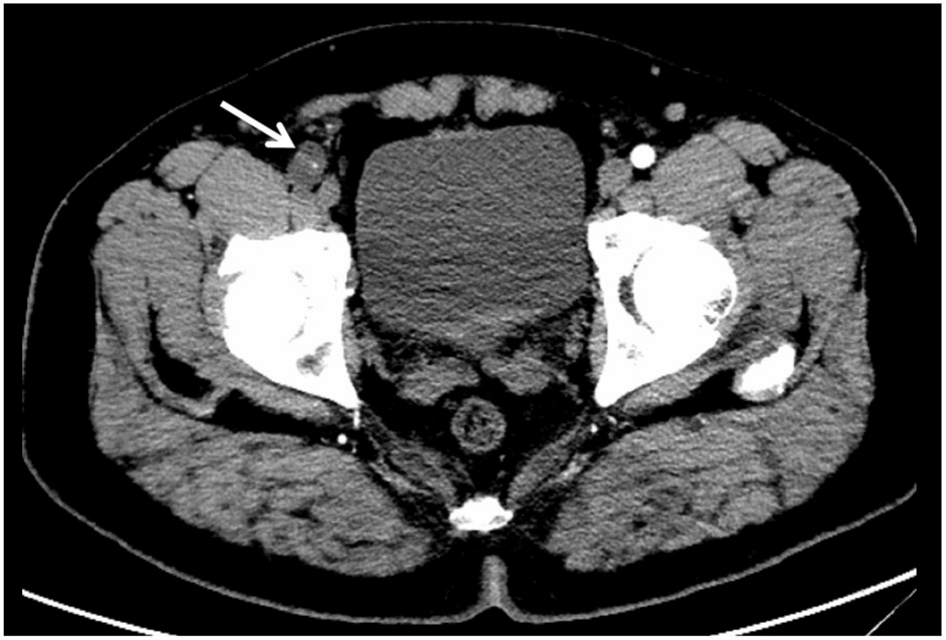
Preoperative, pelvic, contrast-enhanced CT imaging showed a low-density shadow next to the common femoral artery, and the common femoral artery was almost completely occluded (white arrow).
